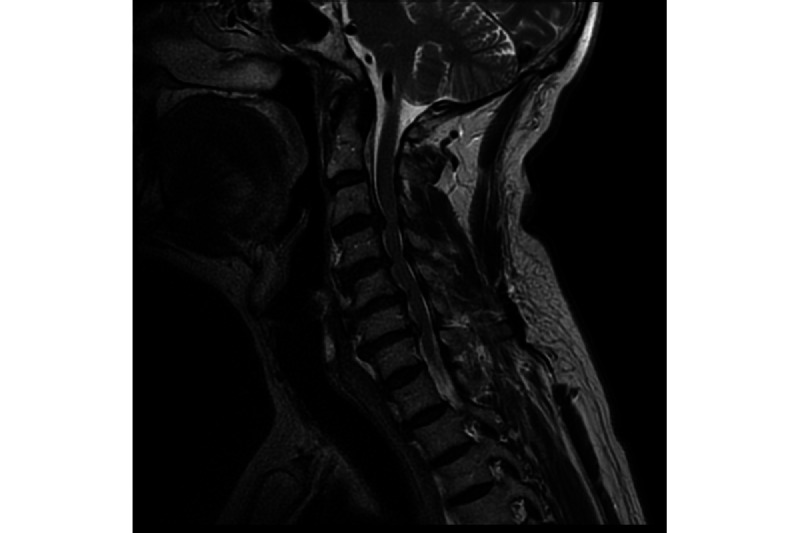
T2-weighted sagittal MRI demonstrating the progression of multilevel degenerative changes in the cervical spine, including disc degeneration at C3-C7.
